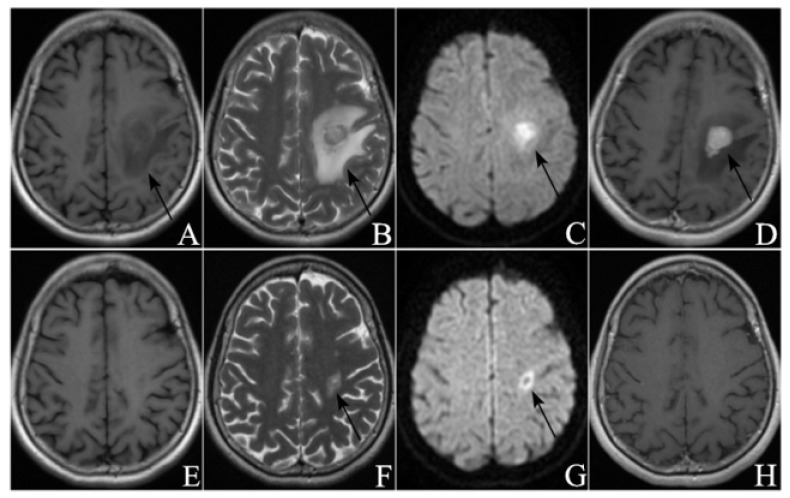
A sixty-five-year-old male PCNSL patient received consolidation WBRT after initial MTX chemotherapy, and the PFS1 was 12.3 months. KPS of this patient was 70 at first relapse. (A–D) were MRI images before HD-MTX rechallenge, and (E–H) were MRI images 60 days after treatment ((A,E) from T1WI, (B,F) from T2WI, (C,G) from DWI, (D,H) from CE-T1WI). The tumor was located in the left frontal lobe and showed homogeneous enhancement before treatment. After treatment, no abnormal enhancement appeared on CE-T1WI, and this patient was judged as CR.
